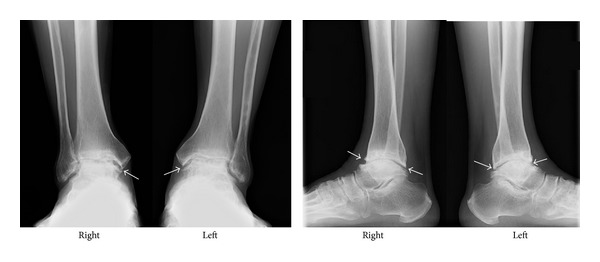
Preoperative radiographs of both the ankles. Arrows show osteophytes. Joint spaces are decreased. No pathological changes around the epiphyses plate are observed.
